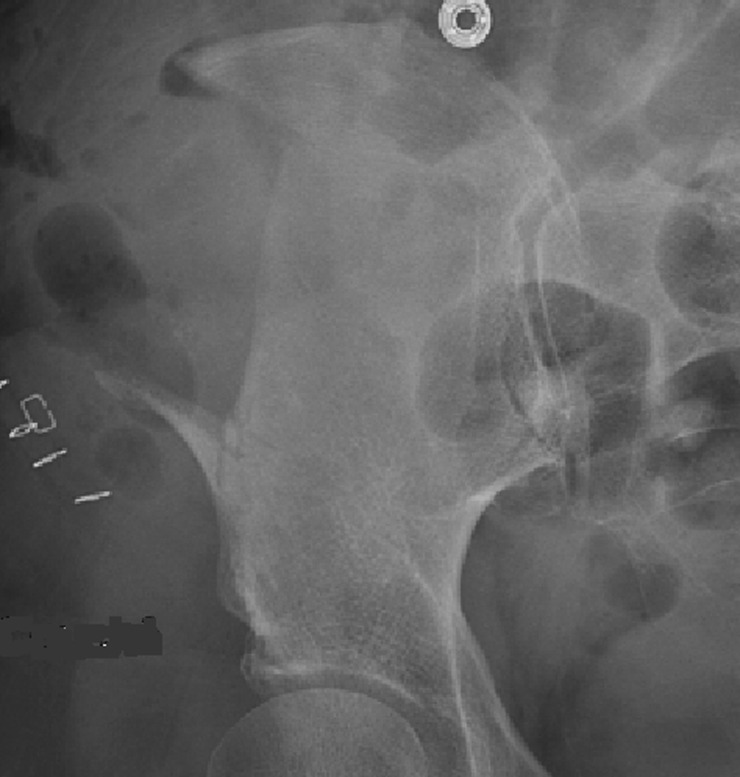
postoperative X-ray showing the partial pelvectomy and complete removal of the lesion
